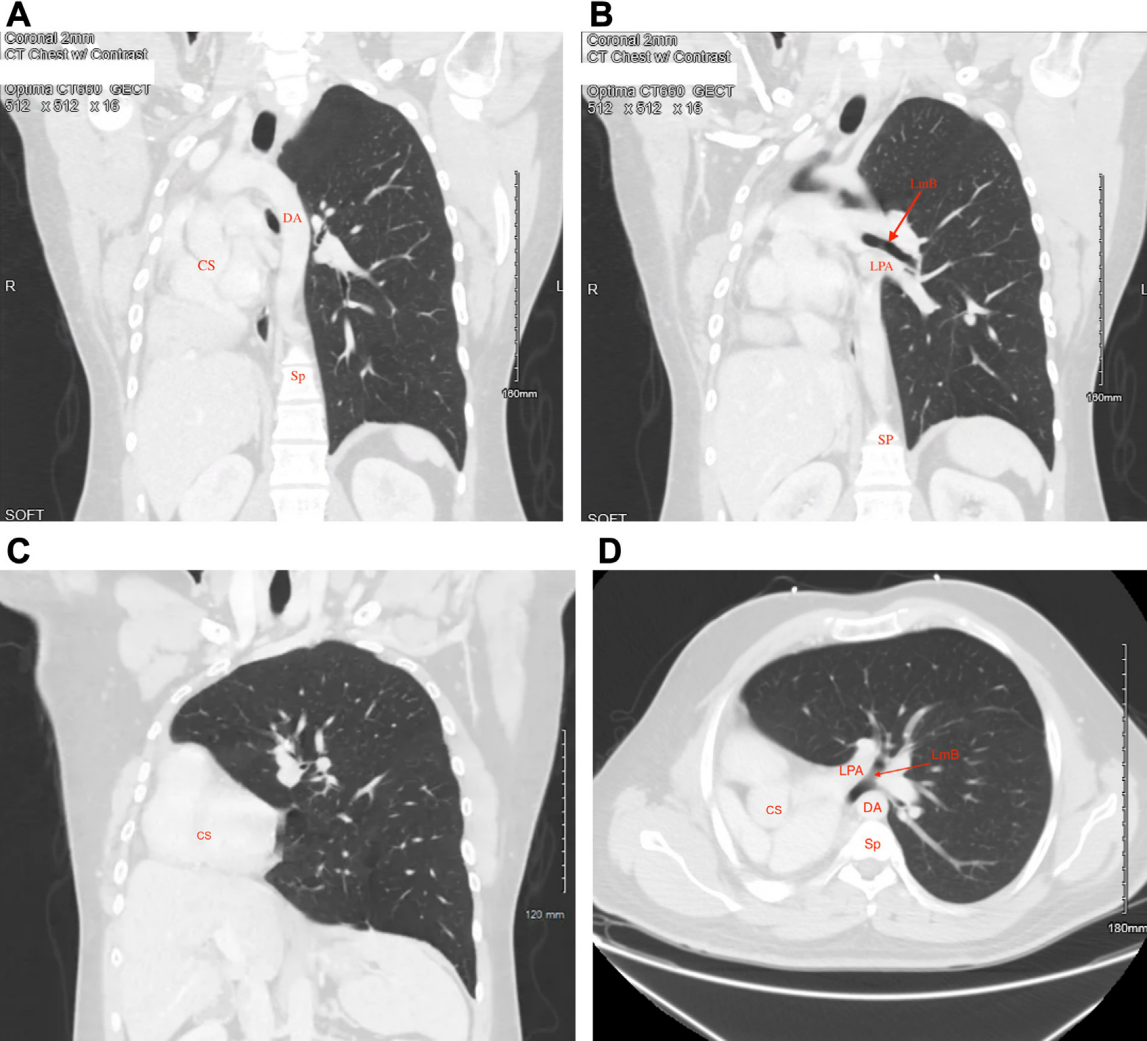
Computed Tomography With Intravenous Contrast of the Chest Showing Mediastinal Changes in Postpneumonectomy Syndrome (A-C) Coronal lung windows. (A) Herniation of the cardiac silhouette (CS) and segment 7 of the liver into the right hemithorax accompanied by supradiaphragmatic pleural thickening and a counterclockwise rotation of the aorta, at the level of the descending aortic arch (DA). (B) A patent left mainstem bronchus (LmB) and left pulmonary artery (LPA) shifted in a clockwise rotation around the aortic fulcrum. (C) Sequela of a remote right pneumonectomy with complete deviation of the mediastinum into the right hemithorax and hyperinflation of the left lung. (D) Axial view of the LmB traveling anterior to the thoracic spine (Sp) and posterior to the LPA.
